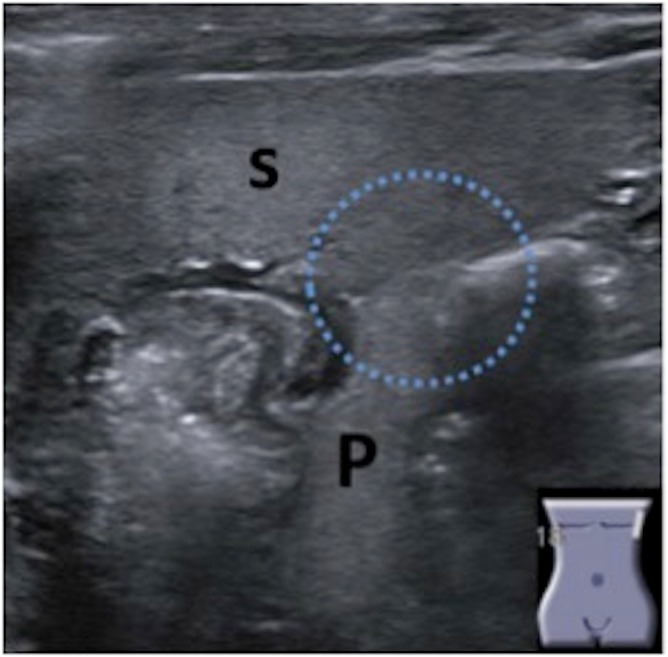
An investigation of the PLG in 20 healthy term newborn infants showed a PLG of 0 on ultrasound with direct contact of the pancreatic tail with the splenic border.
